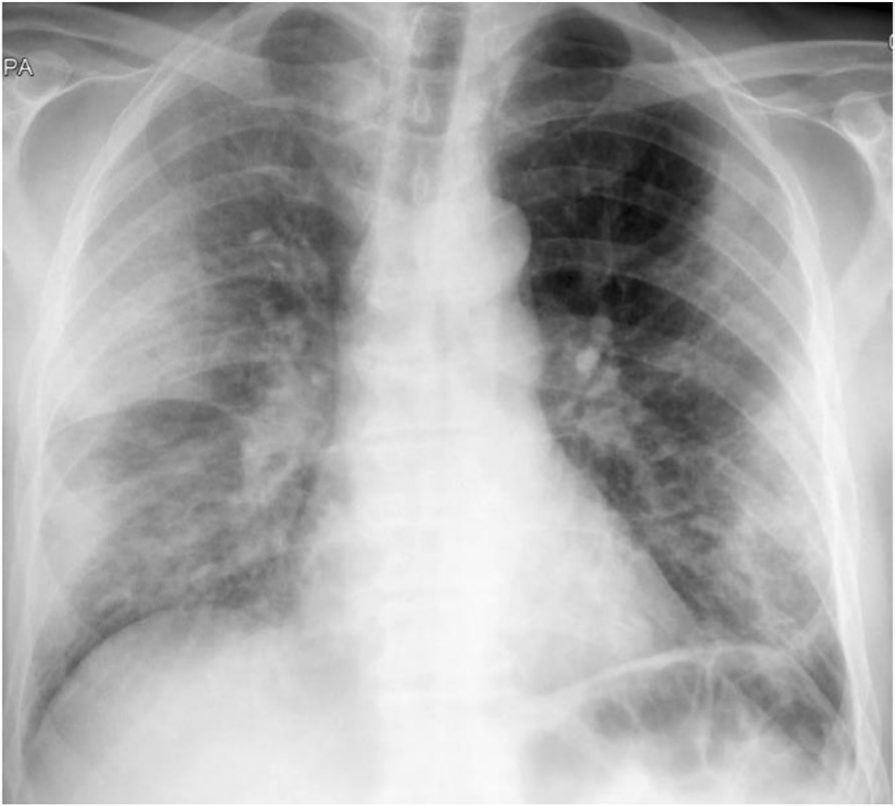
Chest X-ray on admission to the Infectious Diseases Ward which revealed the suspicion of bilateral pneumonia and the symptom of a frosted glass
